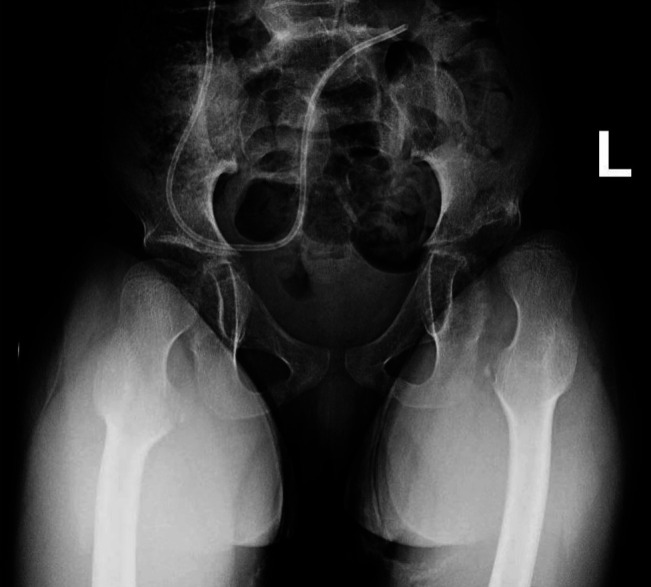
At time of hospital admission, anterior-posterior radiograph of the pelvis revealing worsening left hip subluxation with a Reimers migration index of 80%.
